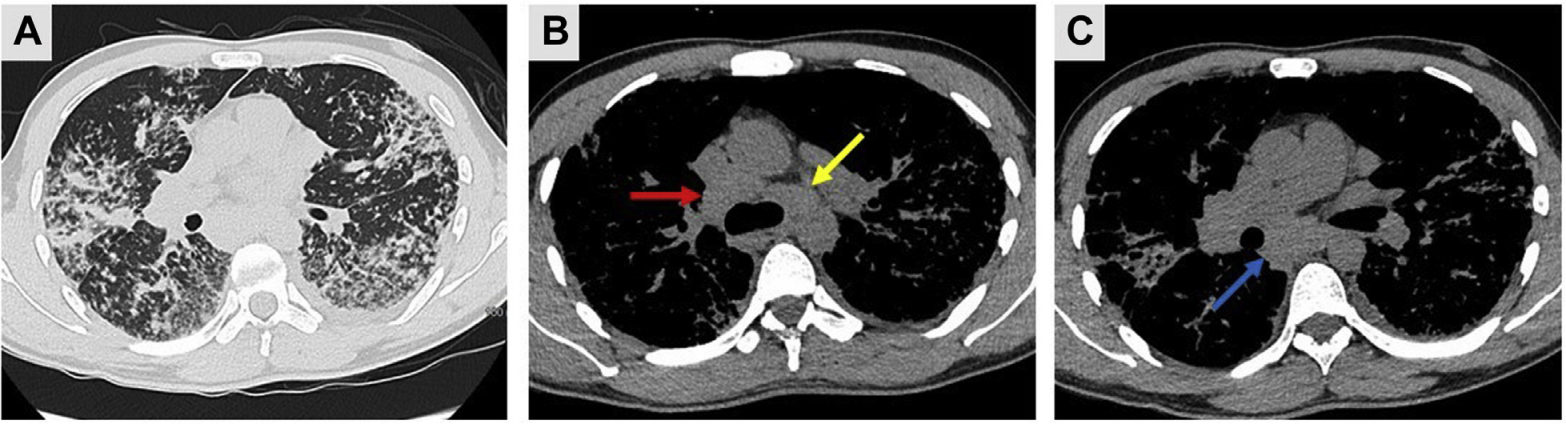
Cross-sectional imaging. A, CT scan of thorax (lung window) showing lung parenchymal changes. B, C, Non-contrast–enhanced CT scan of thorax (mediastinal soft tissue window) showing multiple enlarged (up to 2.8 cm) noncalcified thoracic lymph nodes involving stations 4R (red arrow), 4L (yellow arrow), and 7 (blue arrow).
